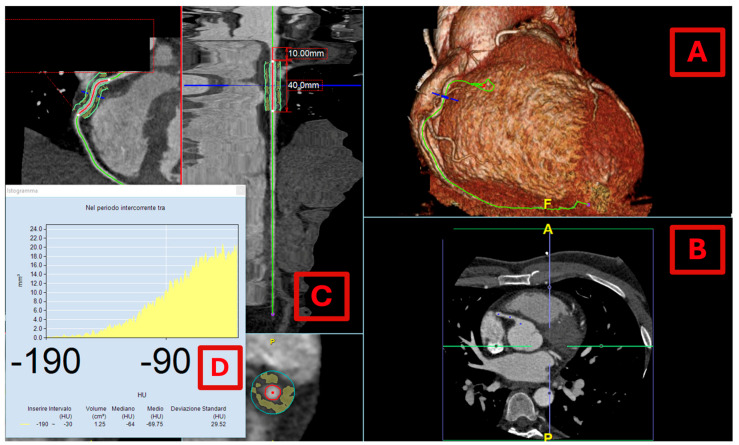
Pericoronary fat attenuation index (pFAI) measurement: Example of how to measure the pericoronary adipose tissue in a male patient with ACS using Aquarius Workstation version 4.4.13.P4 (TeraRecon Inc., Foster City, CA, USA). (A) Three-dimensional (3D) coronary CT reconstruction focused on right coronary artery. (B) Selection of the right coronary artery (RCA) and its region of interest. (C) Pericoronary tissue analysis is performed starting 10 mm from the ostium then the proximal 40 mm segment of the RCA is traced. The adipose tissue within a radial distance from the outer vessel wall equal to the diameter of the vessel is defined perivascular fat. (D) Histogram showing the distribution of pericoronary tissue values in the range of −190 and −30 HU. In Table 1, the main advantages and disadvantages of imaging methods are presented.
